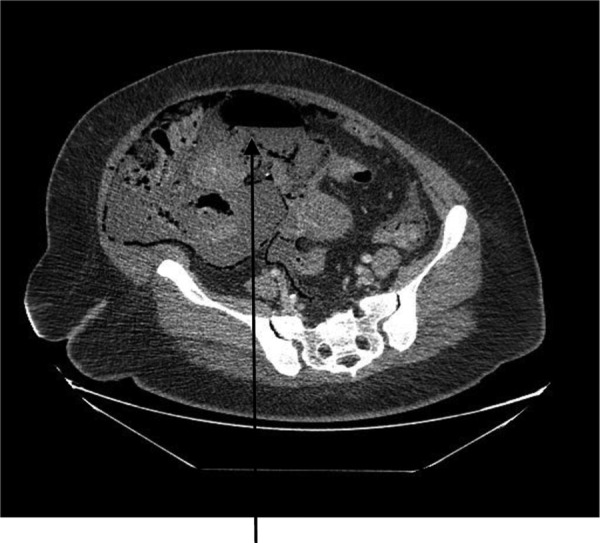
CT abdomen showing large air-filled right abdominal mass that appears to be a septic ovarian cyst.
